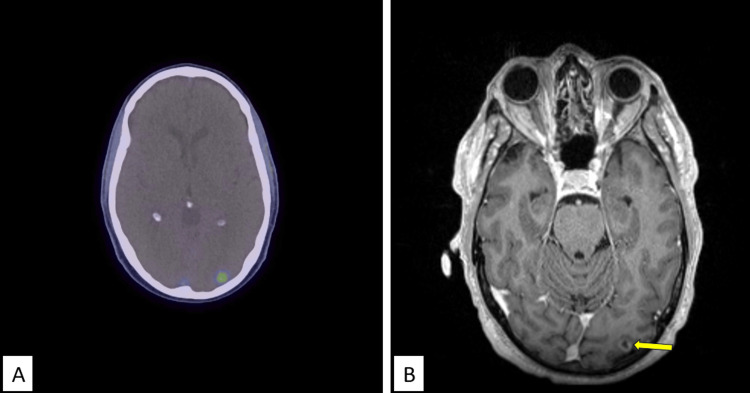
Brain metastatic prostatic adenocarcinoma visualized on [68Ga]PSMA PET/CT and double-contrasted MRI (A) Local PSMA activity fusing to the left occipital lobe peripherally. (B) High-resolution gadolinium double-contrast MRI of the brain revealed a solitary 8.5 mm × 7.0 mm × 9.5 mm peripherally enhancing parenchymal lesion (yellow arrow) with surrounding vasogenic edema. [^68^Ga]PSMA PET/CT, ^68^Gallium prostate-specific membrane antigen PET-CT; PSMA, prostate-specific membrane antigen
